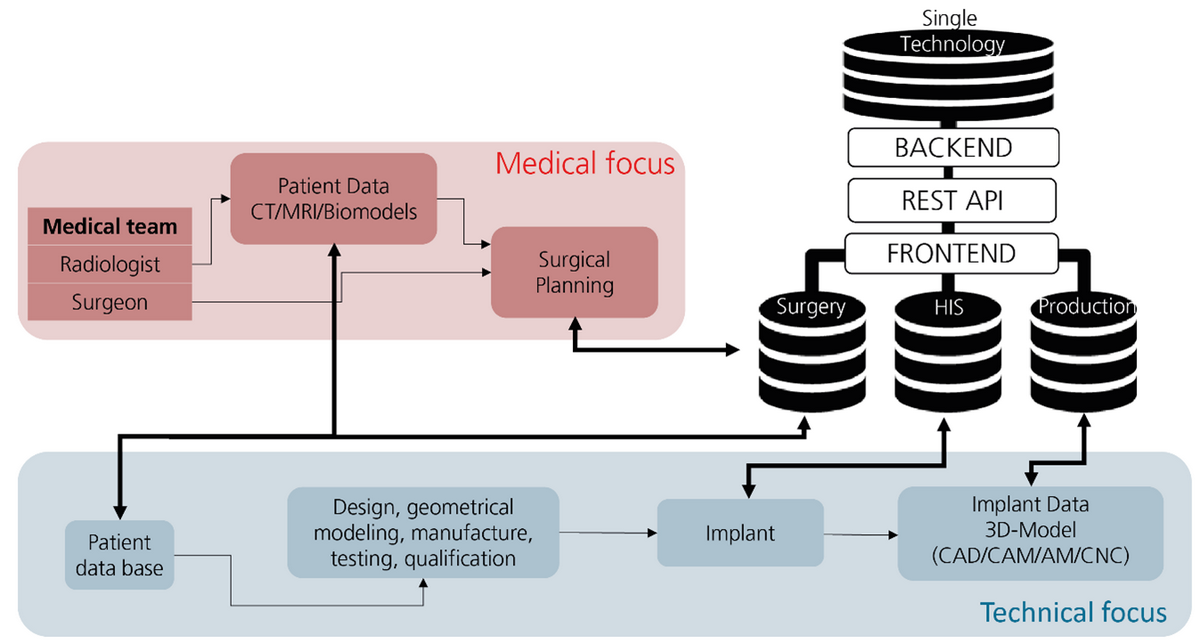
Partial networking of distributed data and information from different domains. AM: additive manufacturing; API: application programming interface; CAD: computer-aided design; CAM: computer-aided manufacturing; CNC; computer numerical control; CT: computed tomography; HIS: hospital information system; MRI: magnetic resonance imaging.
